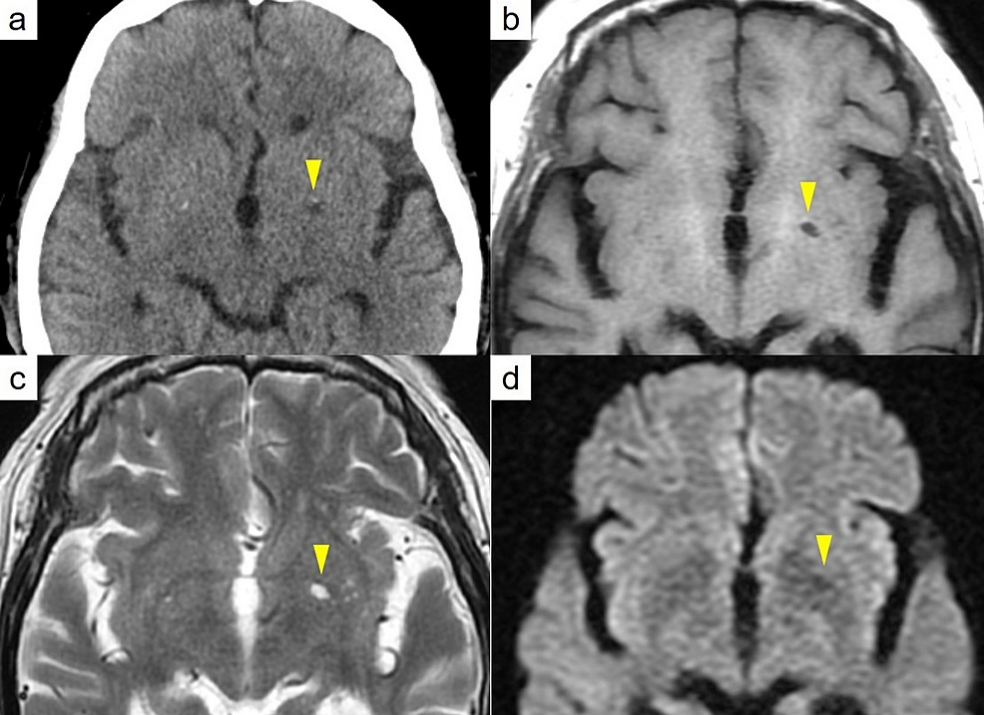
Radiographic images. a. Head CT showed no brain hemorrhage, but hypo-densities on the left globus pallidus. b. Head MRI: On T1 imaging, hypo-intensities on the left globus pallidus. c. On T2 imaging, hyper-intensities on the left globus pallidus. d. On  Diffusion-weighted imaging, hypo-intensities on the left globus pallidus.
